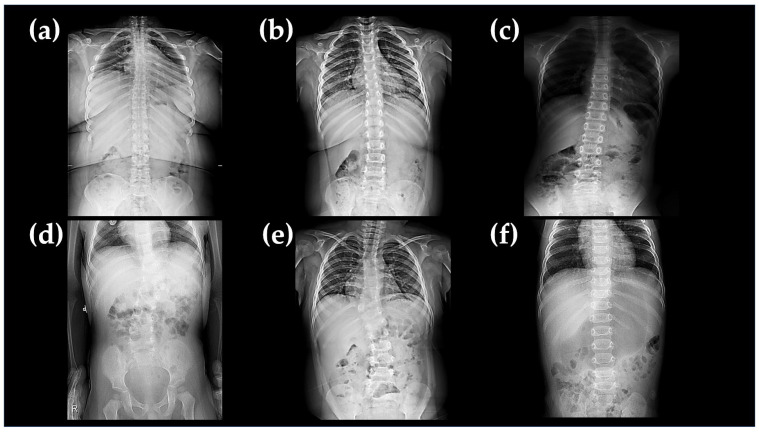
Radiologic findings indicate vertebral abnormalities, including scoliosis and vertebral dysplasia, in Korean patients with 16p11.2 microdeletion syndrome. Three patients, A-III-1 (a), A-III-4 (b), and B-II-2 (c), exhibit scoliosis, while two patients, D-II-1 (d) and E-II-1 (e), present with vertebral dysplasia. (f) Patient F-II-1 had a normal lumbar spine X-ray.
